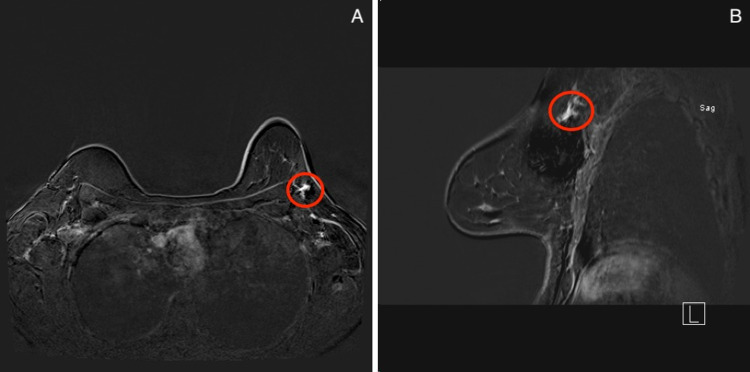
MRI of the left breast. Axial (A) and sagittal (B) T1-weighted maximum-intensity-projection contrast-enhanced with subtraction image shows an irregular shaped mass with heterogeneous enhancement, measuring 18 × 17 × 12 mm in the upper outer aspect of the left breast at the 1:00 position and 13 cm from the nipple (red circles). MRI: magnetic resonance imaging.
